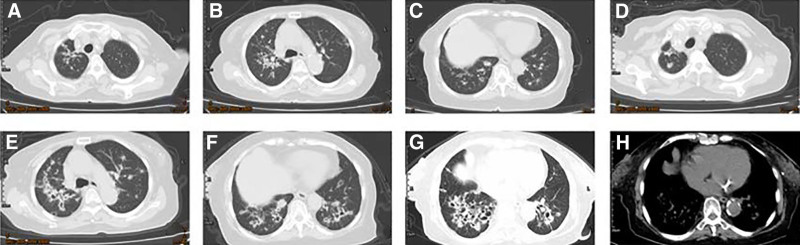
Imaging findings. (A–C) The patient’s early chest CT. (D–F) A chest CT during a period of worsening symptoms despite repeated anti-infection treatment, showing an increase in the lesion size compared to previous scans, with multiple patchy shadows and nodules with cavities in both lungs. (G, H) During hospitalization, after anti-infection treatment, the chest enhanced CT showed no significant change. CT = computed tomography.
